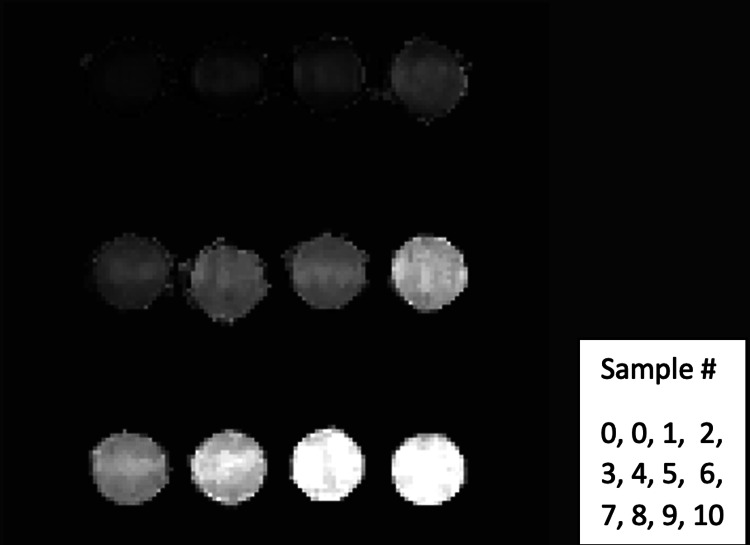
R2* mapping from MRI multi-echo gradient sequence MRI: magnetic resonance imaging
